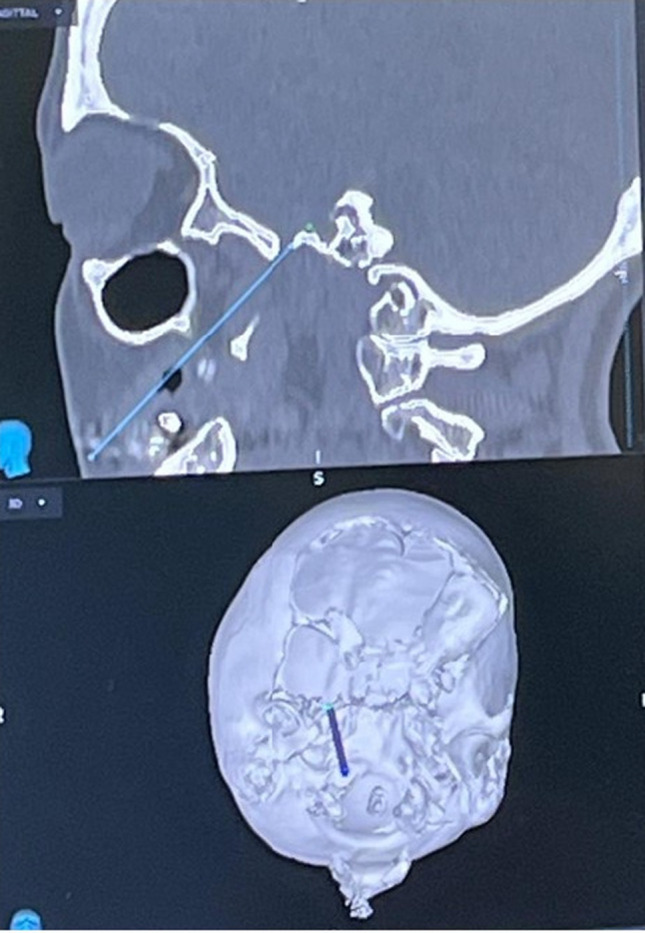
Intraoperative neuronavigation view showing real-time confirmation of the needle trajectory toward the foramen ovale. Sagittal CT reconstruction and 3D surface rendering allow precise alignment of the planned and actual paths, improving accuracy and reducing the need for multiple needle repositionings
